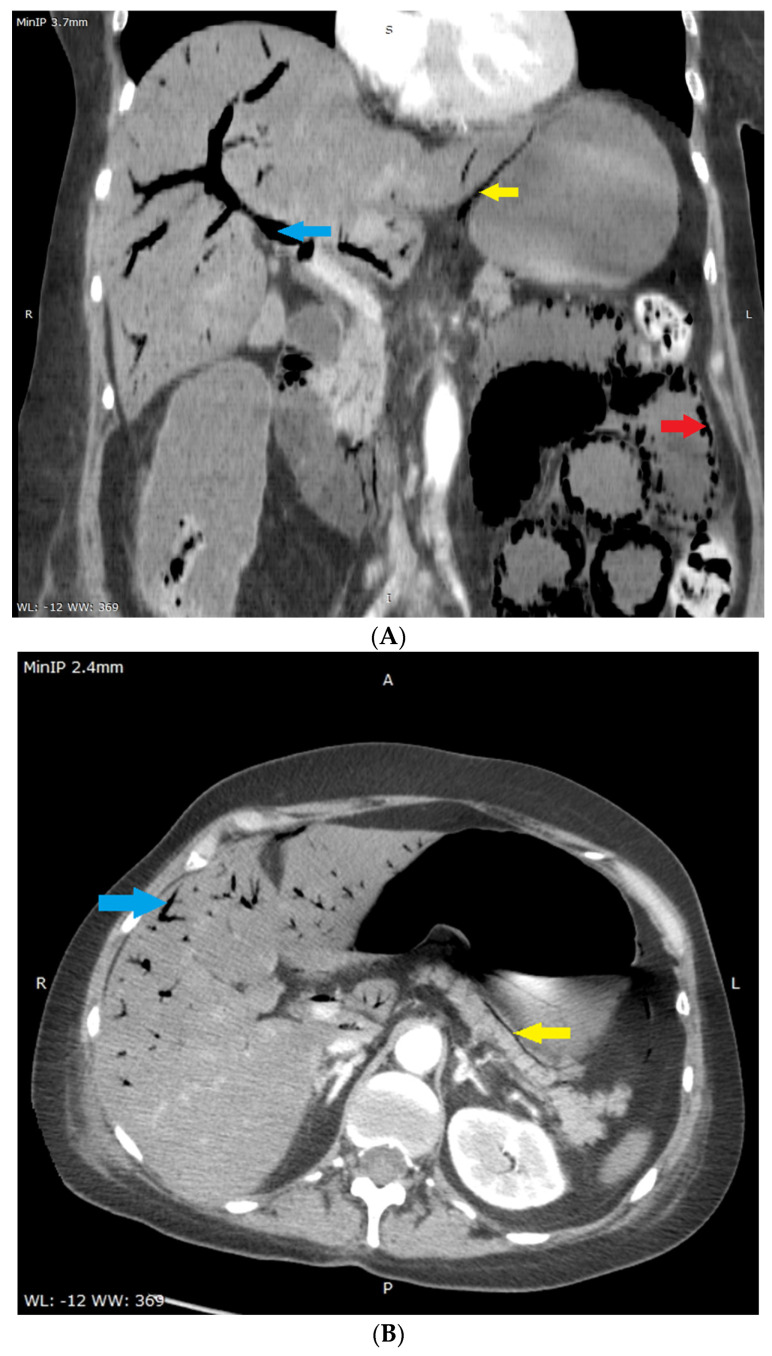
(A,B) Multi-phase abdominal computed tomography performed immediately after liver US. No oral contrast was administered due to urgency–the stomach is filled with accidental content making it isodense to the liver parenchyma. Therefore it is difficult to distinguish this from liver parenchyma in (A). Routine amount of intravenous contrast agent was administered. (A)–coronal reconstruction, portal-venous phase, slice showing the portal vein was selected, minimum intensity projection with 3.7 mm thickness was used in order to better show gas in the portal circulation. Visualized are: gas in the wall of the small intestine (red arrow), gas in the portal vein (blue arrow) and most of its branches-on the picture visible mostly in segments V and VIII, gas in the wall of stomach-lesser curvature (yellow arrow), and distended small intestine filled with fluid (width 33 mm). (B)–axial plane, portal-venous phase, at the level of pancreas, adrenal glands and portal vein, minimum intensity projection with 2.4 mm thickness was used for the same reason as in the (A). Visualized are: gas in the portal vein (visible gas-blood level) and most of its branches (blue arrow), paring of hepatic segment VI portal branches due to anti-gravitational distribution, gas in the wall of stomach (yellow arrow). No gas was present in the hepatic vein circulation as an aquarium sign [7], the celiac trunk and superior mesenteric artery were narrow but patent, there were single gas microbubbles present in the spleen and in superior mesenteric vein, intra- and extrahepatic ducts were not dilated, and no pneumobilia was present.
